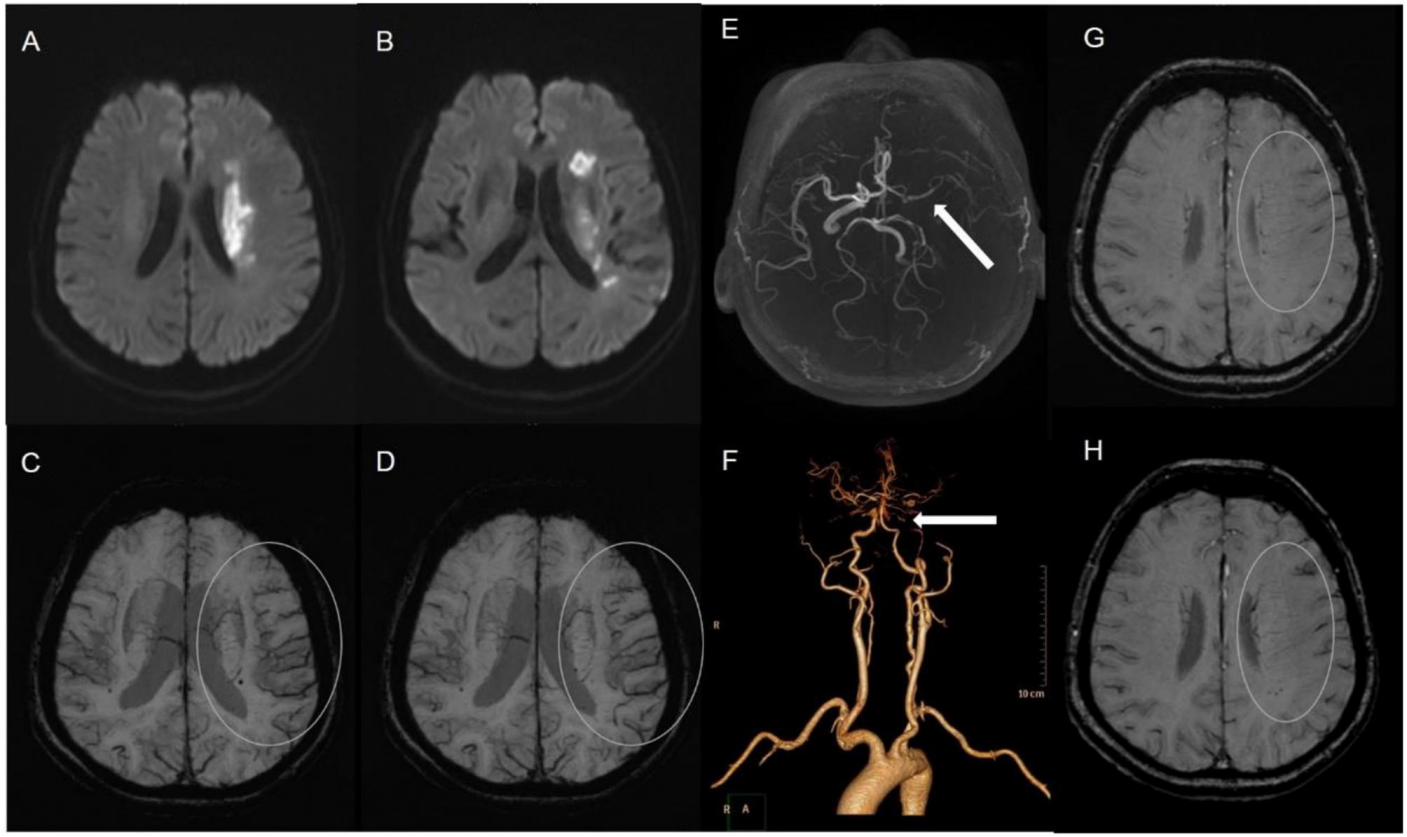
A 62-female patient with left CWI (A,B). SWI indicates significantly more and larger vessels with a greater signal loss (APCV) in the left hemisphere [(C,D) white circle]. CTA showed left internal carotid artery occlusion [(F) white arrow]. The status of cerebral arterial circle was graded as good with the presence of contrast filling from the M1 to M3 of MCA and anterior cerebral artery [(E) white arrow]. The SWI showed prominent medullary veins hypointense signals (APMV) in the left hemisphere [(G,H) white circle].
